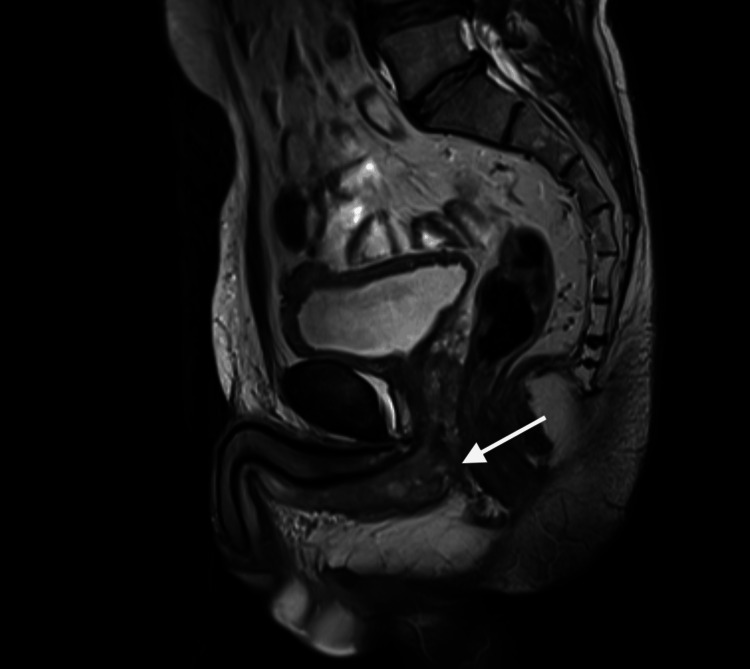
Urethral mass on MRI saggital view (white arrow).
